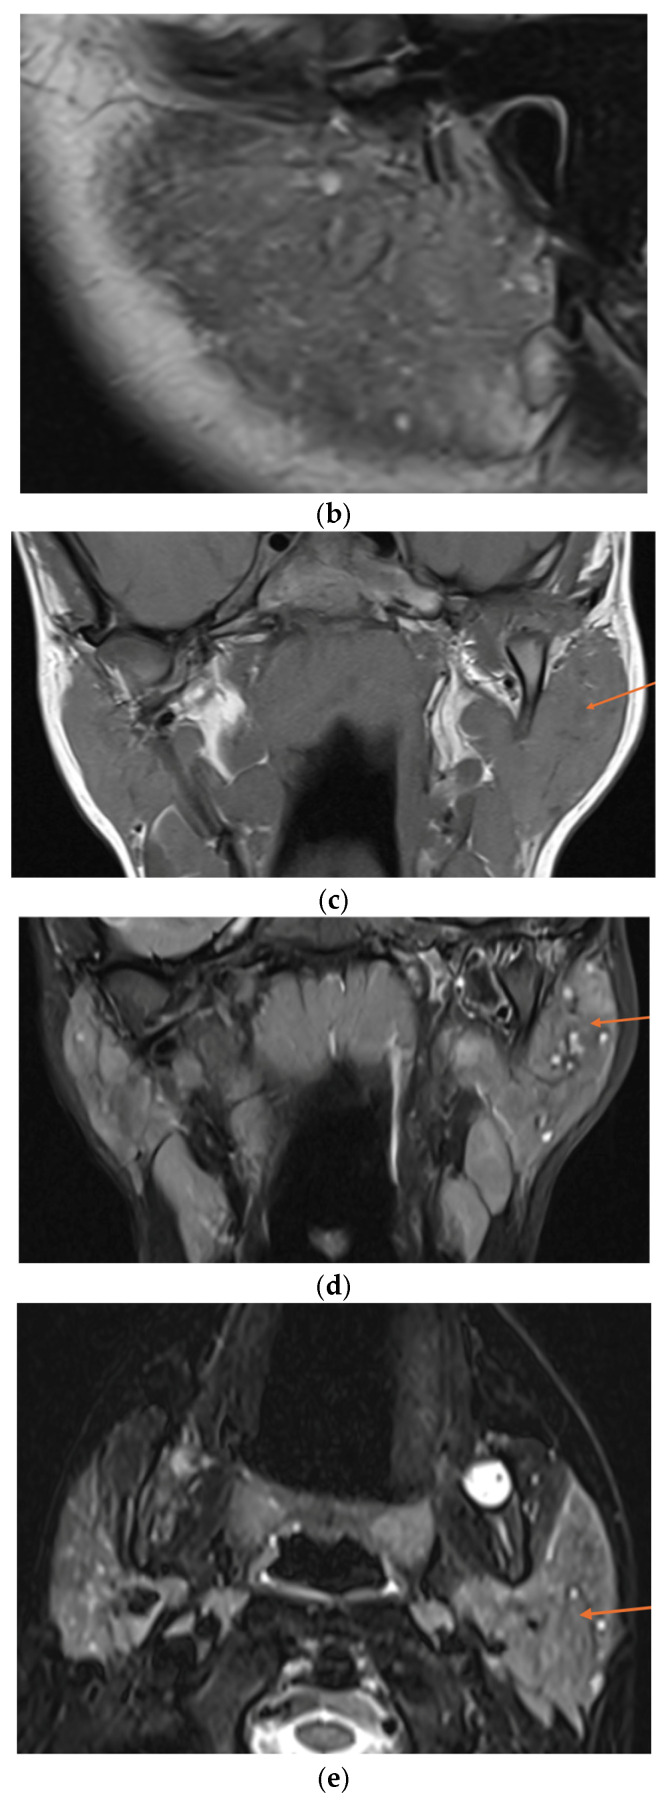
Cervical MRI in patient 15. There is enlargement of both parotid glands, more pronounced on the left. The glands measure 47 × 20 × 11 mm (CC/LL/AP) on the left and 46 × 16 × 10 mm (CC/AP/LL) on the right. Both glands show a heterogeneous structure with T2-hyperintense fluid-like components, cystic in nature, measuring less than 1 cm. Imaging includes sagittal T2 (left and right sides) (a,b), coronal T1 (c), STIR (d), and axial STIR (e) sequences. The arrows indicate the left parotid gland.
